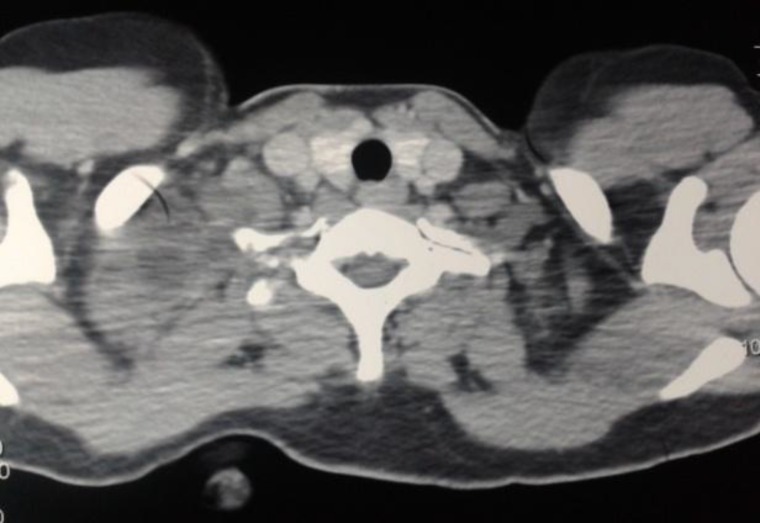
Contrast Enhanced CT image showing erosion of transverse process of T1 vertebra and 1st rib with maintained fat planes, by heterogenous enhanced lesion
